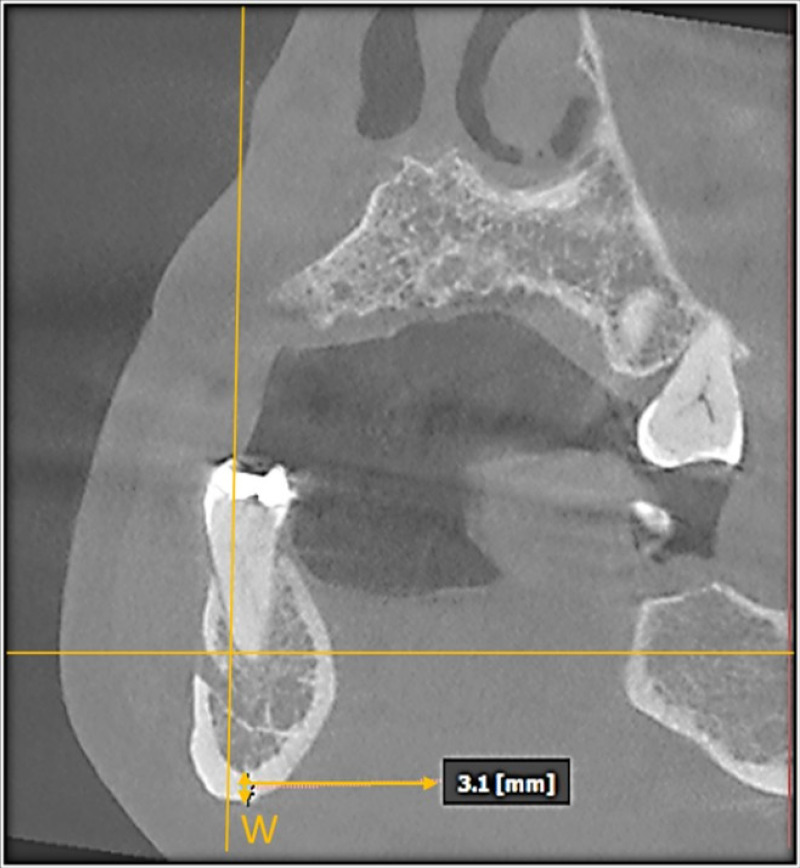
CTMI - computed tomography mental index.
